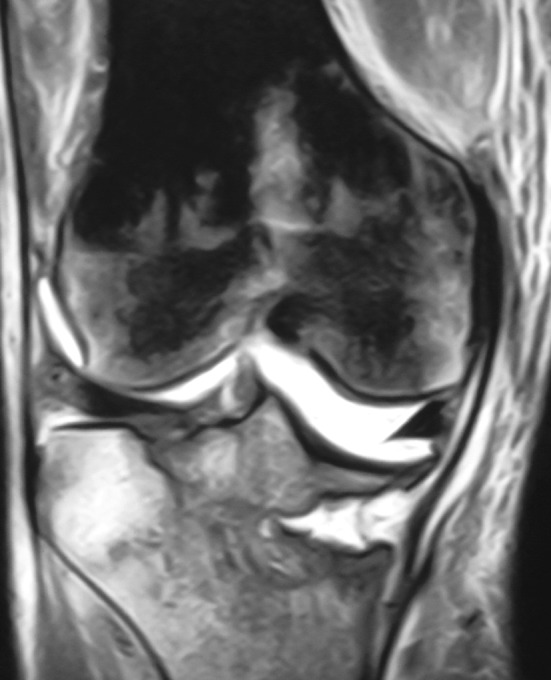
Magnetic resonance imaging (MRI) of the right knee before the operation. The magnetic resonance imaging of both knees showed bilateral tibial plateau fracture on medial side and dense bone marrow edema around, constitutional signal changes on distal femurs, edema on soft tissues around the knee, and effusion on both knees
